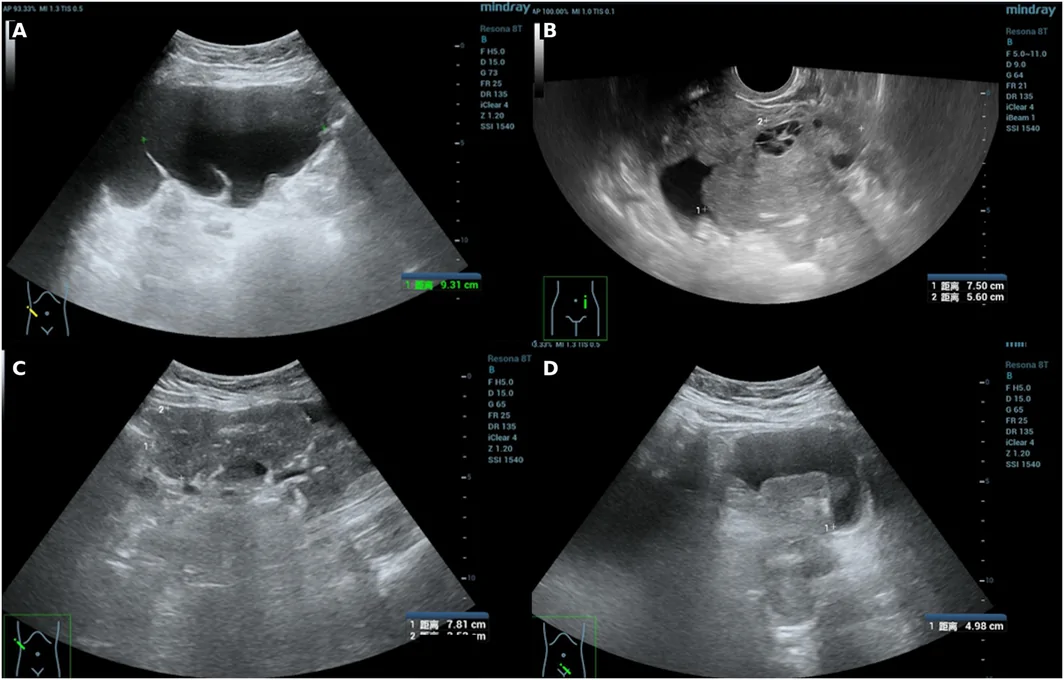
Ultrasound on August 22, 2024 (A–D). Pre‐treatment transvaginal ultrasound reveals complex bilateral adnexal masses, multiple hypoechoic lesions on the omentum and bowel serosa, and abundant abdominopelvic free fluid.
